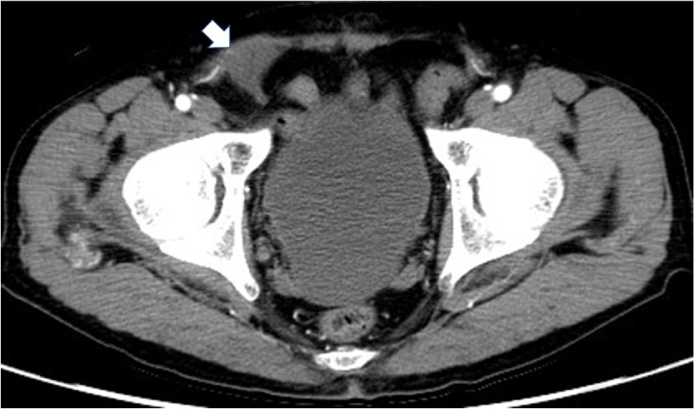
Abdominal computed tomography shows a meshoma at the location of the plug (white arrow).
